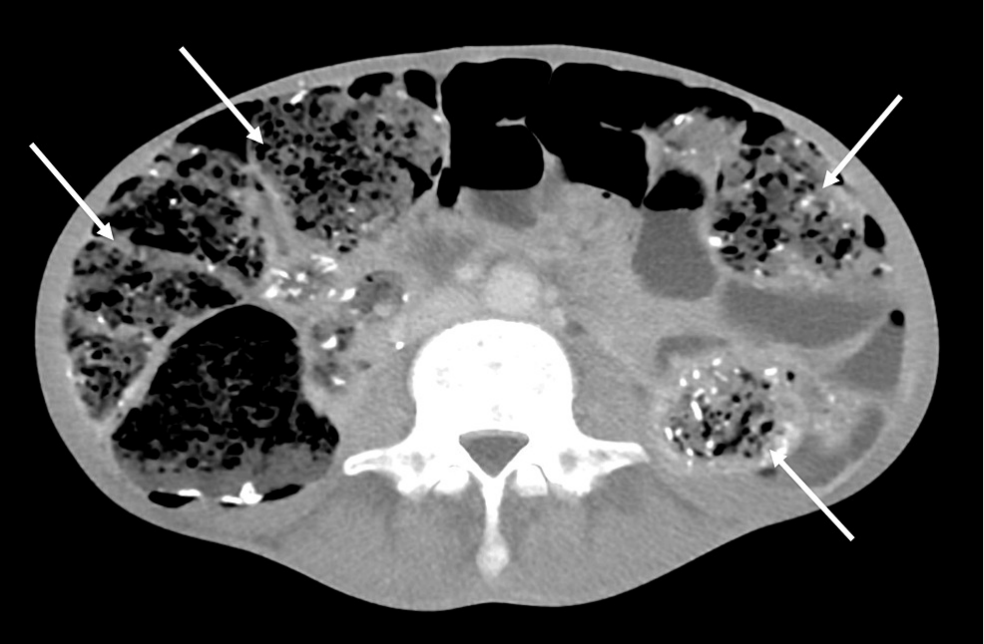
Computed tomography of the abdomen Dilated colon filled with a large amount of feces containing gas and some high-density materials.
